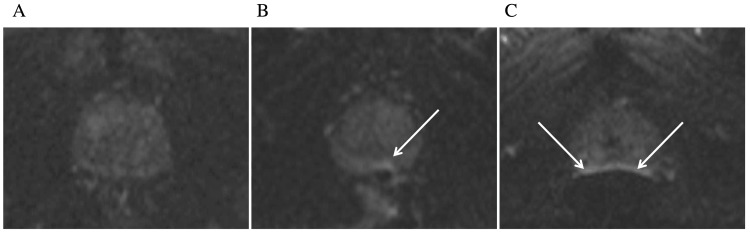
Axial DWI images demonstrate none (A), mild (B), and moderate (C) artefact secondary to rectal gas. DWI: diffusion-weighted imaging
